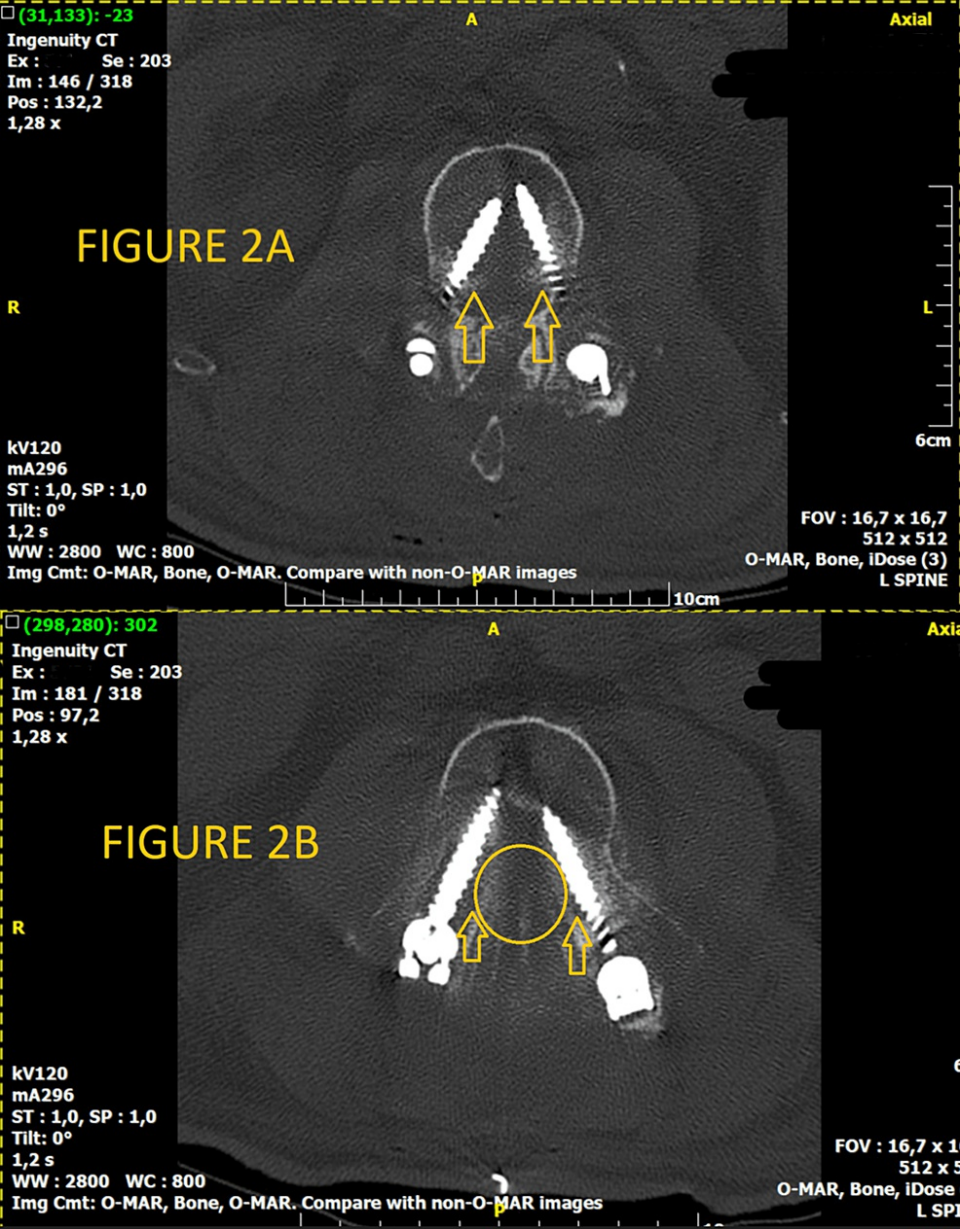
(A) L3 axial view of CT scan; (B) L2 axial view of CT scan Axial view of CT scan with the single-level screw fixation with no mechanical obstruction or cord perforation
